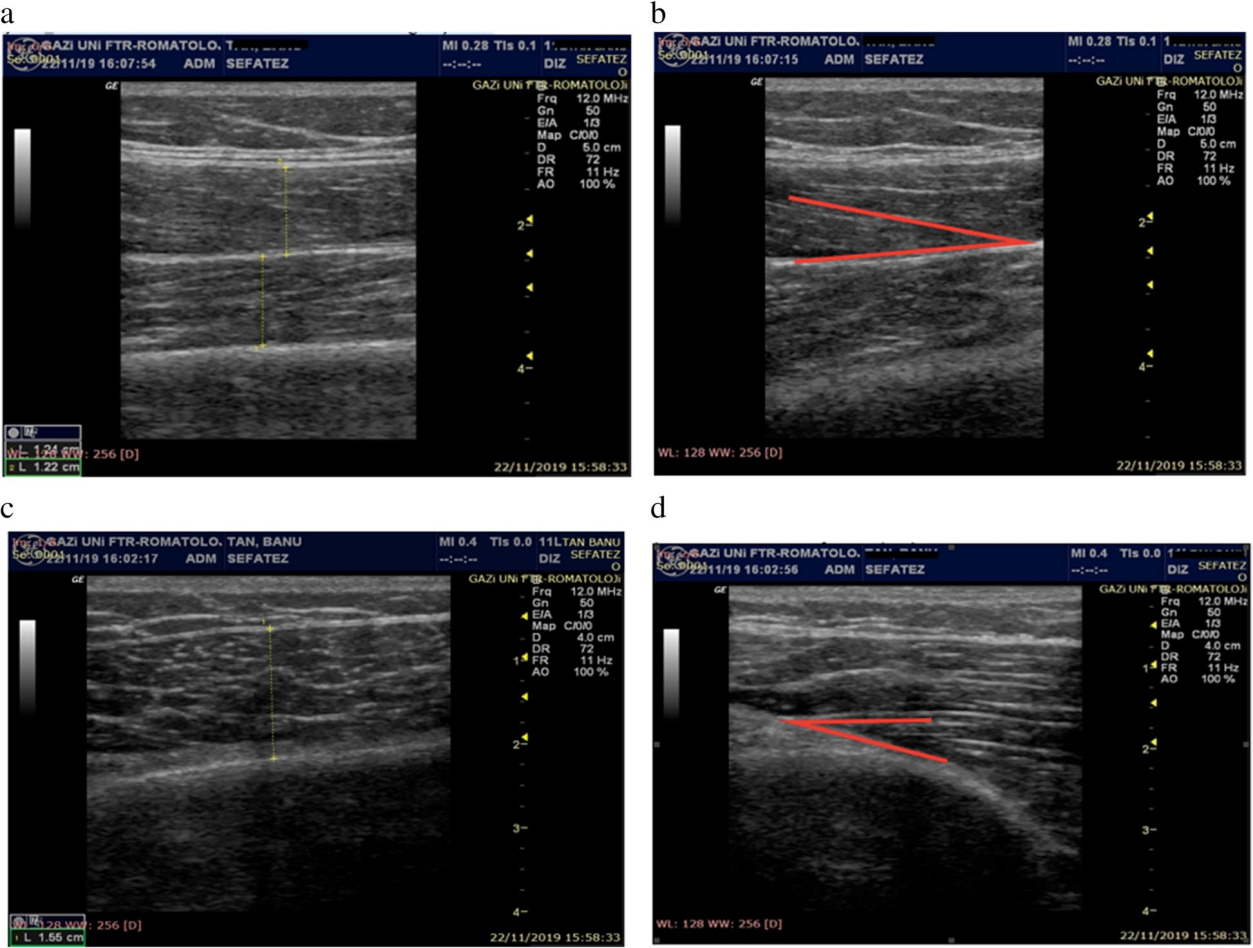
Muscle thickness* and pennate angle measurements. a D1: Rectus femoris muscle thickness, D2: Vastus intermedius muscle thickness, b α: Rectus femoris pennate angle, c D3: Vastus medialis muscle thickness d α: Vastus medialis pennate angle *Muscle thickness: Distance between superficial and deep aponeurosis by holding the ultrasound probe axially and pressing it to the maximum, Pennate angle: the angle formed at the attachment (with its insertion) of the muscle fascicles to the deep aponeurosis
